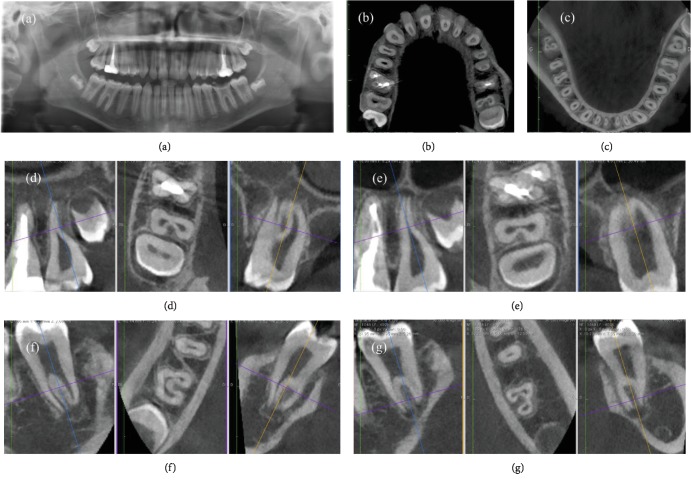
(a) Panorex; (b) CT-image of maxillary arch; (c) CT-image of mandibular arch; (d) CT images of the maxillary right second molar showing the C-shaped anatomy; (e) CT images of the maxillary left second molar showing the C-shaped anatomy; (f) CT images of the mandibular right second molar showing the C-shaped anatomy; (g) CT images of the maxillary left second molar showing the C-shaped anatomy.
